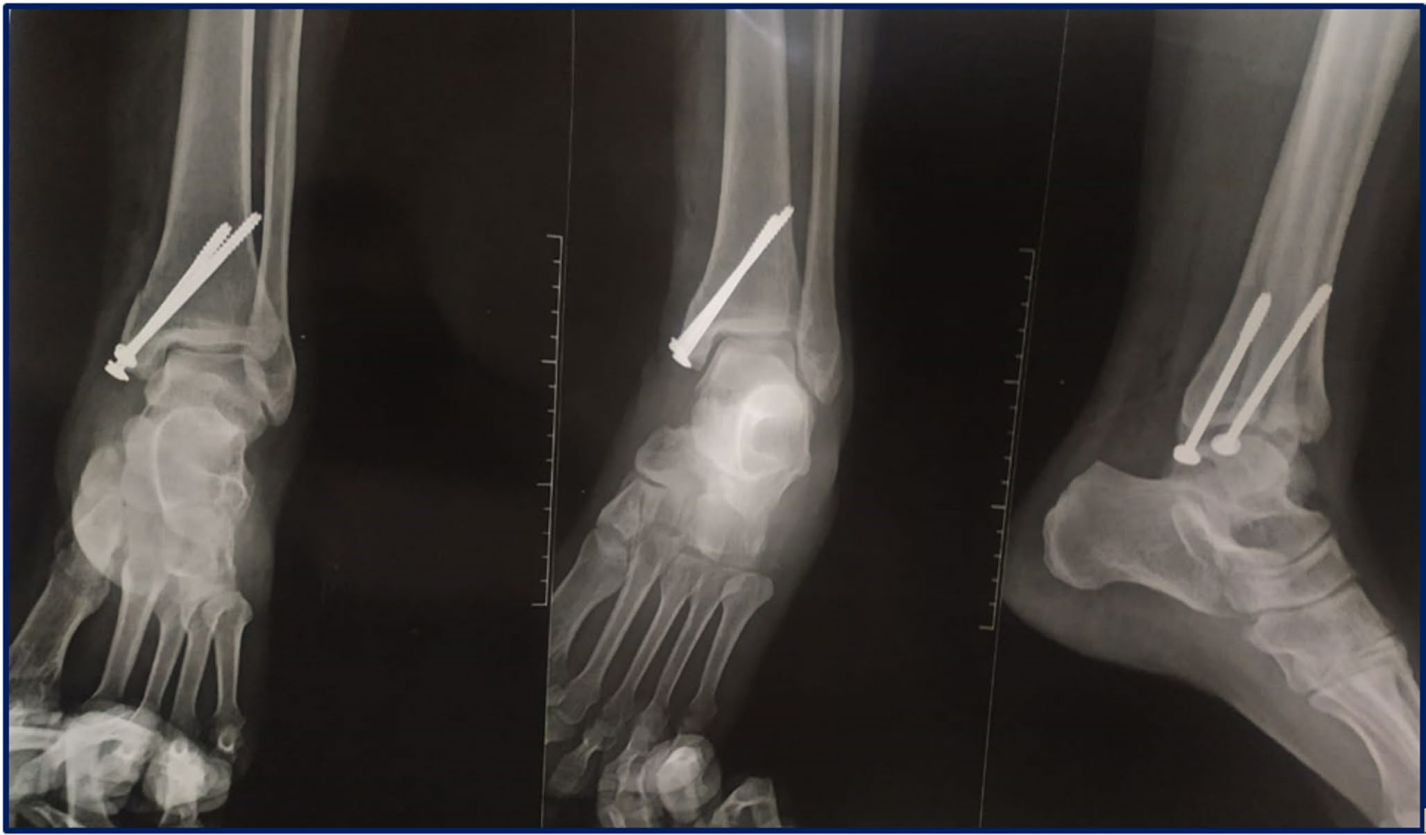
Surgical technique for ORIF (continued): post-operative radiographs
